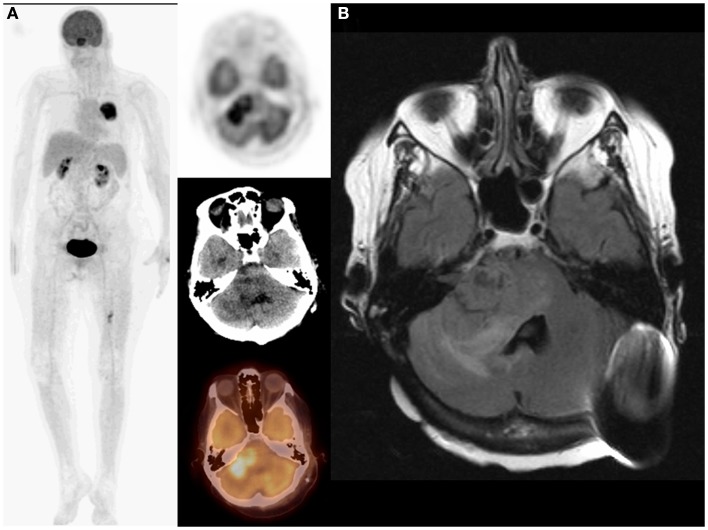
Eighty-year-old female with non-small-cell lung cancer in the right lung status post Cyberknife therapy. On restaging PET/CT: MIP (maximum intensity projection) and transaxial PET, CT, and fused images (A) demonstrated complete response to therapy in the right lung and a new left temporoparietal lobe lesion suspicious for metastasis. PET/CT finding was confirmed on MRI (B). Adding the brain to the imaged field of view changed both staging (I–IV) and management as patient underwent whole brain radiation.
